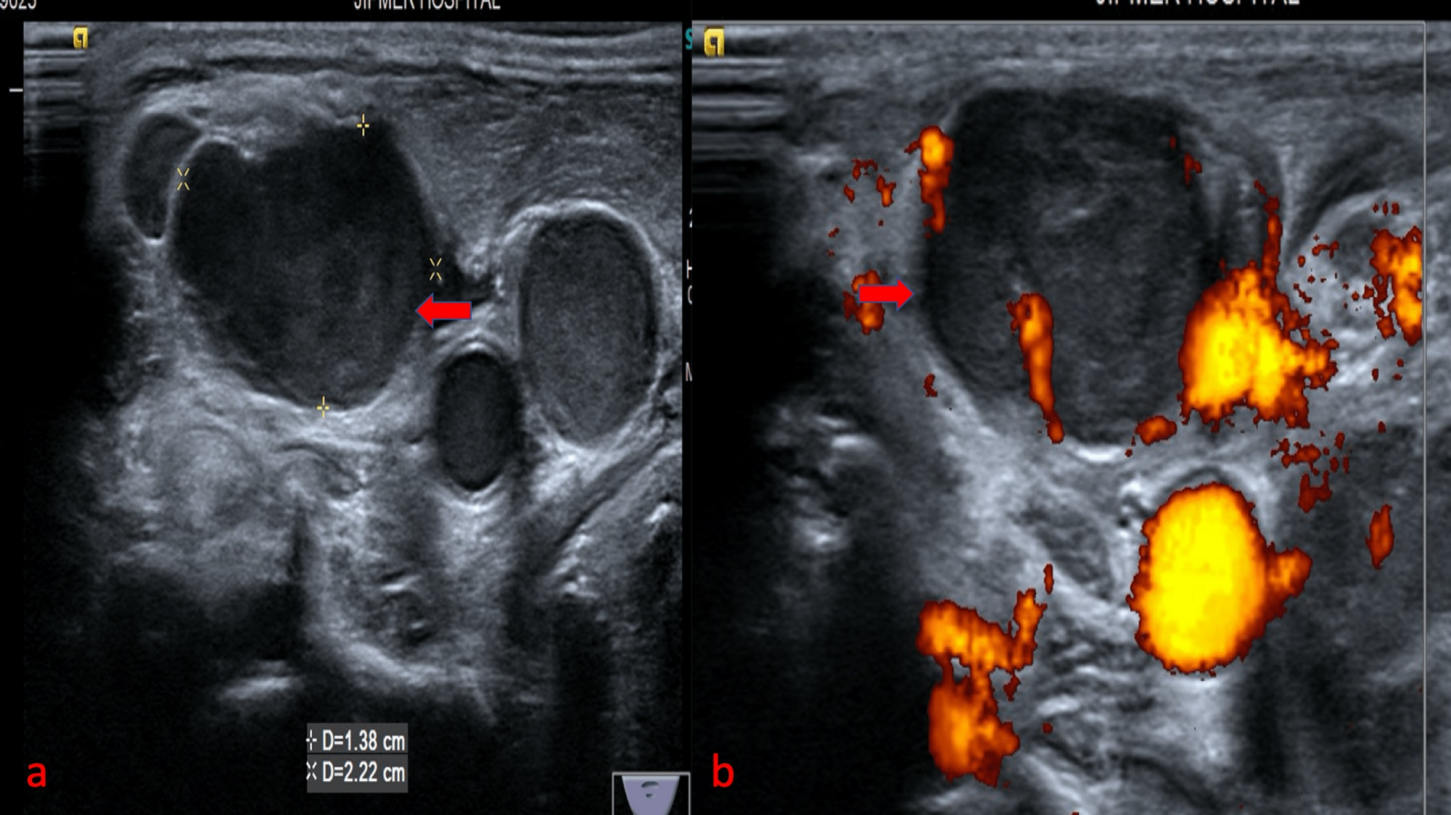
Imaging of tuberculous cervical lymphadenopathy: (a) BMUS shows an enlarged necrotic lymph node adjacent to the common carotid artery (red arrow), (b) CDUS shows the hilar vascularity within the lymph node (red arrow) BMUS: B-mode ultrasound; CDUS: color doppler ultrasound
